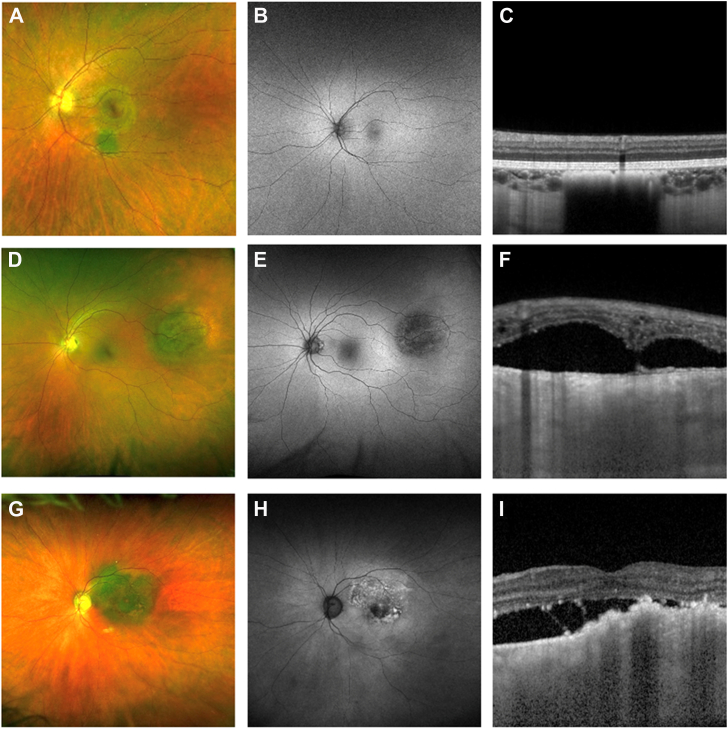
Color fundus photographs, fundus autofluorescence images, and OCTs of 3 cases: (A–C) choroidal nevus with basal diameter 2 mm, no SRF, and flat on USG. D–F, Indeterminate choroidal lesion with basal diameter 5 mm, presence of SRF, and 1.1-mm thickness on USG. G–I, Choroidal melanoma with basal diameter >5 mm, confluent orange pigment, presence of SRF, and 1.6-mm thickness on USG. SRF = subretinal fluid; USG = ultrasonography.
